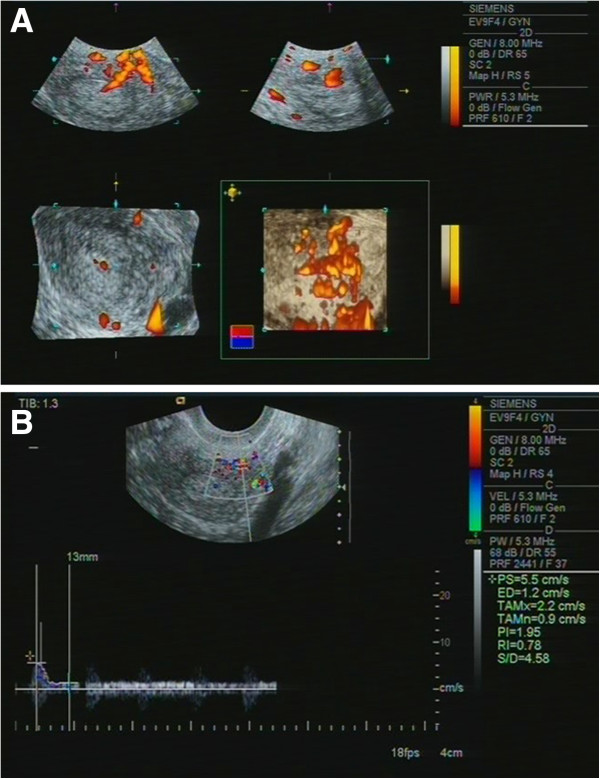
Arteriovenous malformation in the anterior myometrium wall. Hysteroscopy was performed 7 months ago. (A) In a three-dimensional ultrasound angiography on the anterior myometrium wall, the tortuous vascular plexus is determined with interconnected arteries and veins. (B) The blood outflow from the plexus artery to the vein in the endometrium with spectral arterial flow (speed up to 4 cm/s) changed by venous flow (up to 1 cm/s) while transducer was kept in fixed position.
